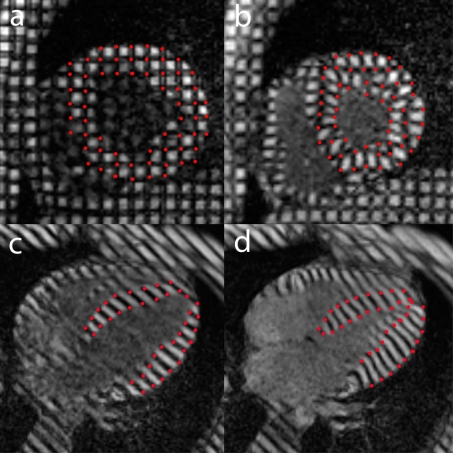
Example of MRI study with 2D tagging analysis. Tagging in cardiac short axis (a,b) and horizontal long axis (c,d), end–diastolic (left) and end–systolic time frame (right). Tracking of the grid intersections *(indicated in red)* on the short axis views, and the intersections of the tags with the endo- and epicardial border *(indicated in red)* on the long-axis views, allow analyzing the local myocardial deformation.
